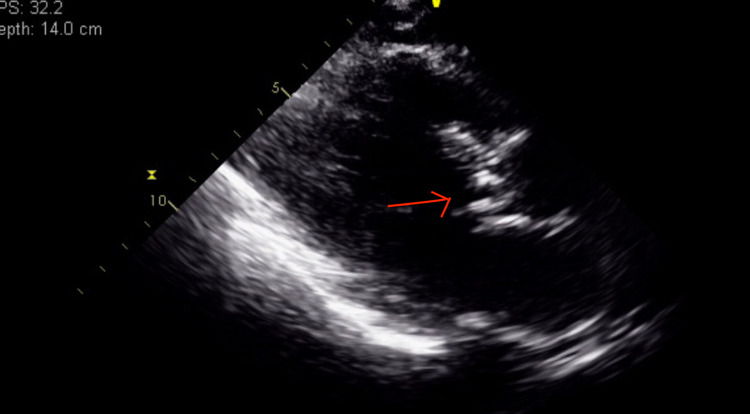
PLAX-vegetations on the right and non-coronary cusps of aortic leaflets on the ventricular side at the start of systole. PLAX: parasternal long-axis view.
